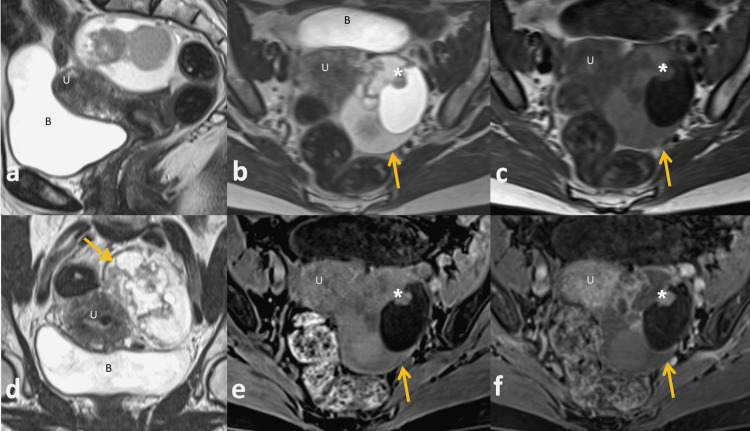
MRI images Sagittal T2 (a), axial T2 (b), axial T1 (c), coronal T2 (d), axial T1 fat-saturation and post-contrast fat-saturation T1 (e-f) . These show a complex multilocular septate left adnexal cyst (arrow) abutting the uterus (U) and posterior to the bladder (B). The cyst contains multiple enhancing septae and an enhancing solid nodule (asterisk). The final pathological diagnosis following surgical excision was an endometrioid adenocarcinoma FIGO stage 1A.
